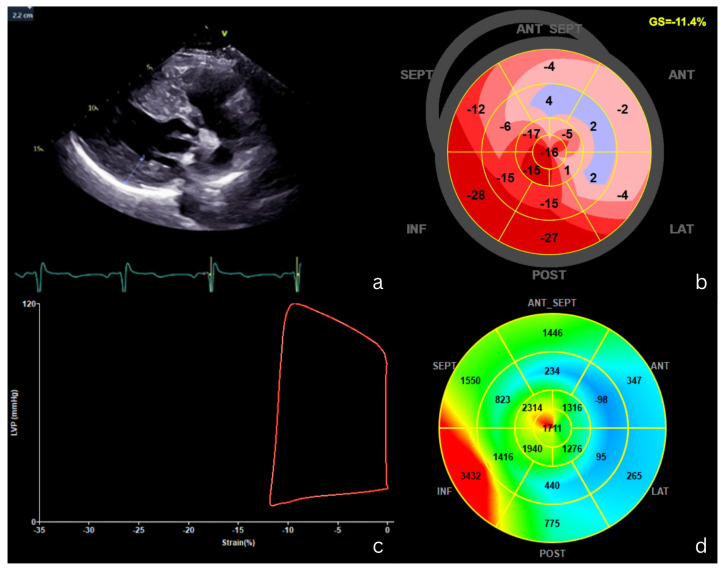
MW analysis in a patient with hypertrophic cardiomyopathy. (a) Parasternal long-axis view demonstrates severe ventricular hypertrophy. (b) Global longitudinal strain bull’s-eye plot shows impaired longitudinal deformation in hypertrophied antero-lateral segments, with a GLS of −11.4%. (c) The pressure–strain loop displays a reduced enclosed area and a rightward shift, indicating marked impairment of myocardial work. (d) The myocardial work index bull’s-eye plot demonstrates reduced myocardial work in the basal antero-lateral segment (light-blue shaded) and increased work in the inferior segment (red shaded). These findings indicate significant regional myocardial dysfunction and heterogeneity, which are associated with higher risk of adverse clinical events, highlighting the potential prognostic value of MW indices in hypertrophic cardiomyopathy. GS, global longitudinal strain; ANT, anterior; LAT, lateral; POST, posterior; ANT SEPT, antero-septal; INF, inferior; SEPT, septal; V, apical view orientation marker.
